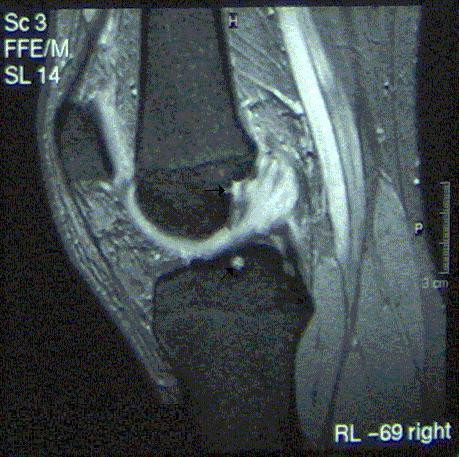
A sagittal T2-weighted MRI showing thickening and pathological appearance of the ACL.
